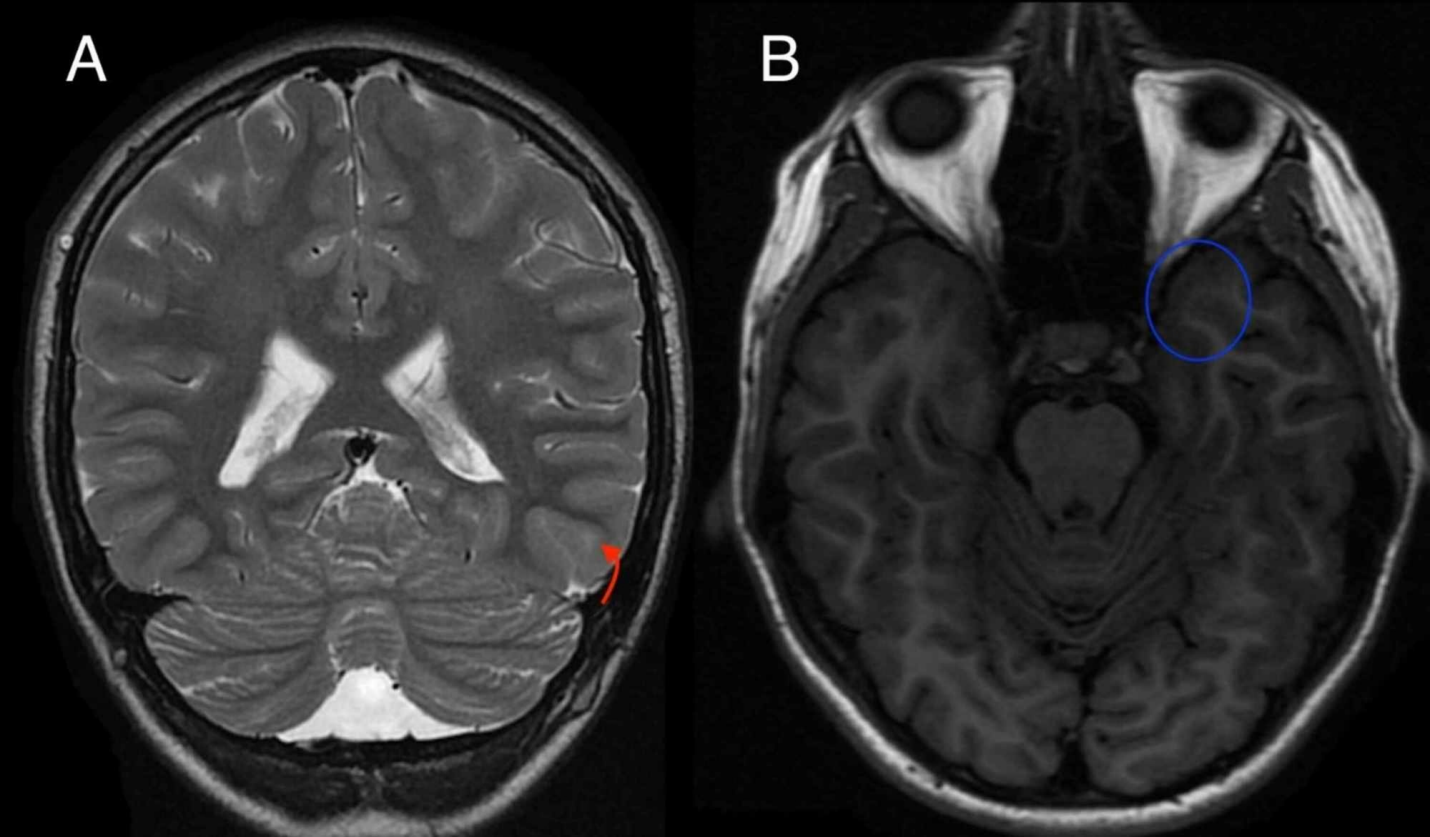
Coronal T2-weighted MR image of the brain (Figure 1A) demonstrates a focus of hyperintensity within the left temporal lobe (curved red arrow). Axial T1-weighted MR image of the brain (Figure 1B) demonstrates mild asymmetry of the hippocampal regions, with slight hyperintensity seen in the left hippocampus (blue circle).
